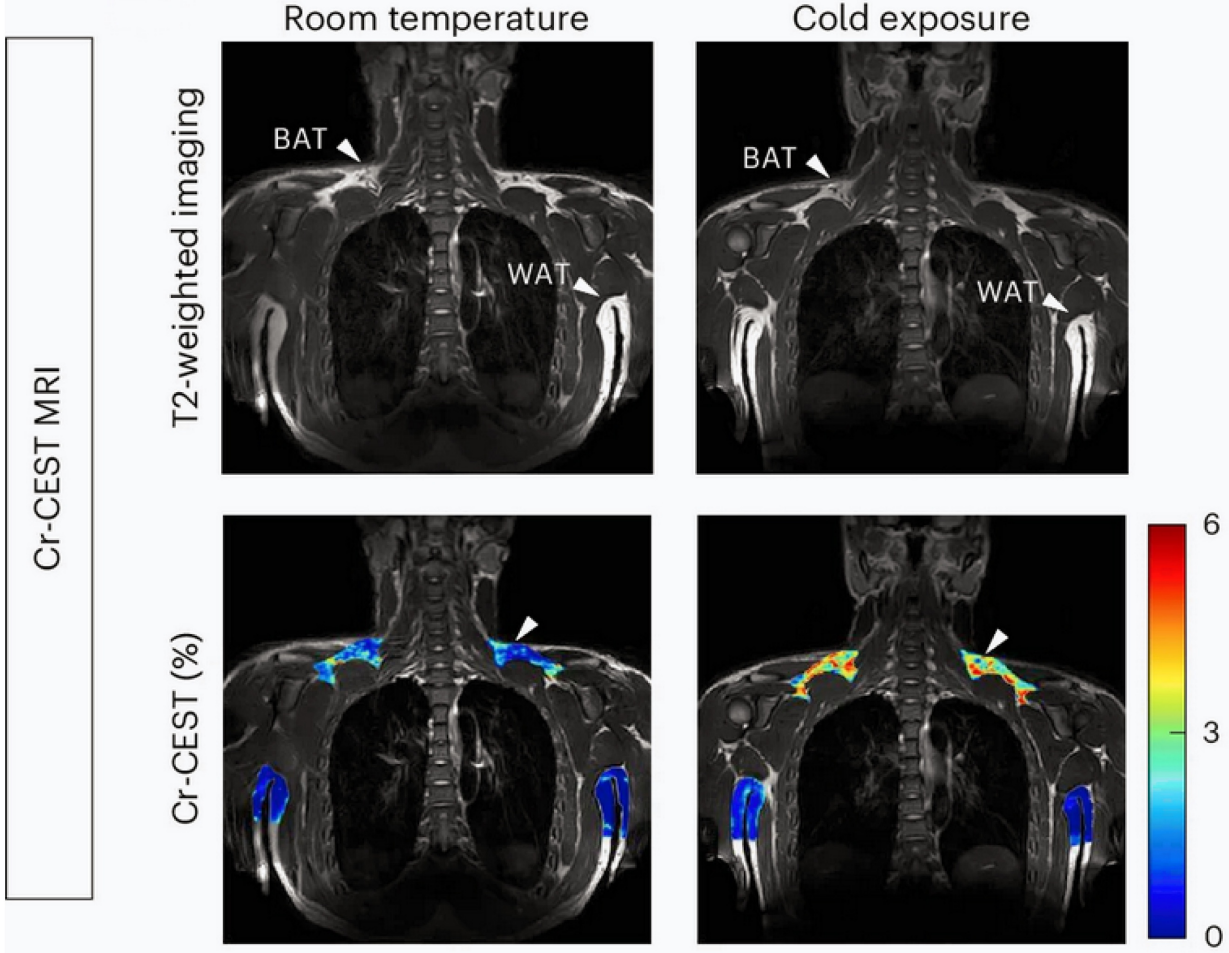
Creatine-based CEST imaging of brown adipose tissue. Brown adipose tissue (BAT) and white adipose tissue (WAT) presented in T2-weighted imaging (upper plane) and creatine-based chemical exchange saturation transfer (CEST) imaging (lower plane). BAT is reflected at room temperature (lower plane, left, white arrow), while the signal of BAT is increased after cold exposure (lower plane, right, white arrow). Figure 4 is reproduced with permission from Springer Nature (Nat Metab. 2024; 6: 1367-79), an open access article under a CC-BY 4.0 license. BAT: brown adipose tissue; CEST: chemical exchange saturation transfer; MRI: magnetic resonance imaging; WAT: white adipose tissue.
